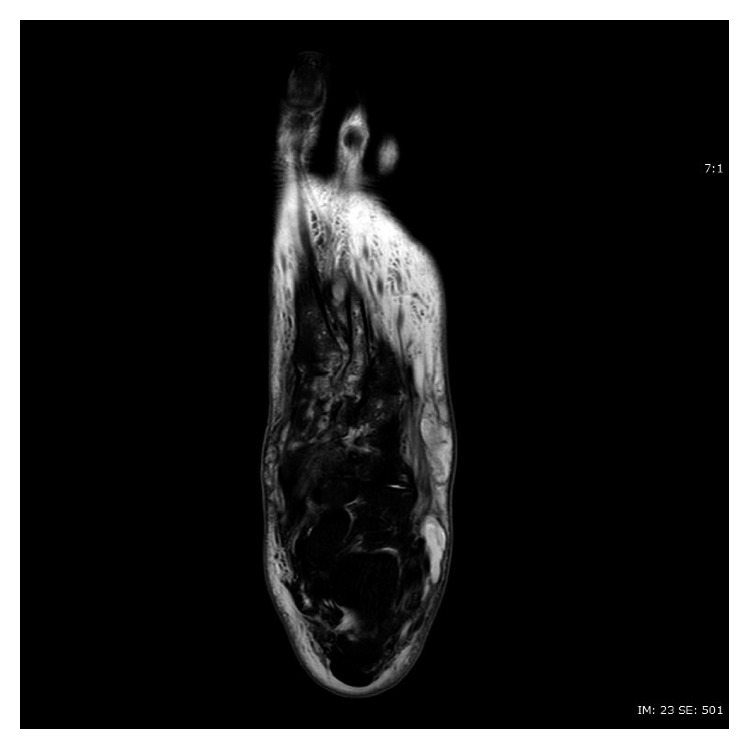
T2-weighted axial image of MRI of left foot without contrast, showing increased signals in soft tissues (compatible with cellulitis), as well as deep-seated multilocular collection at Lisfranc's joints and second metatarsophalangeal joints.
